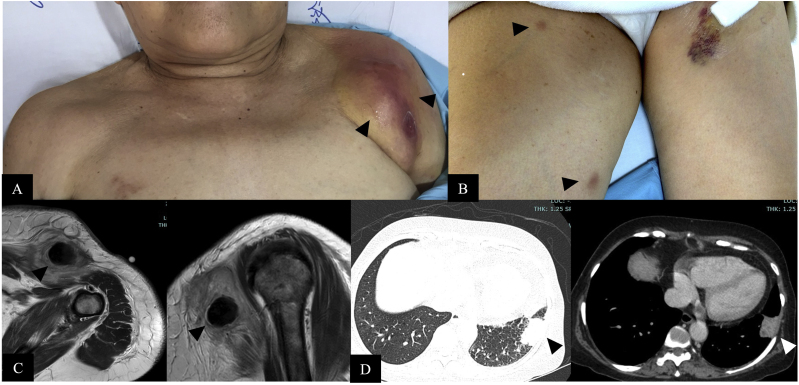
At primary diagnosis, physical examination revealed a large violaceous mass on the left shoulder (A) and painful nodules on both thighs (B). MRI showed multiple enhancing soft tissue abscesses at the left shoulder (C), and CT scan of chest revealed a heterogeneous, enhancing mass in the left lower lung (D), consistent with invasive *Aspergillus fumigatus* infection.
